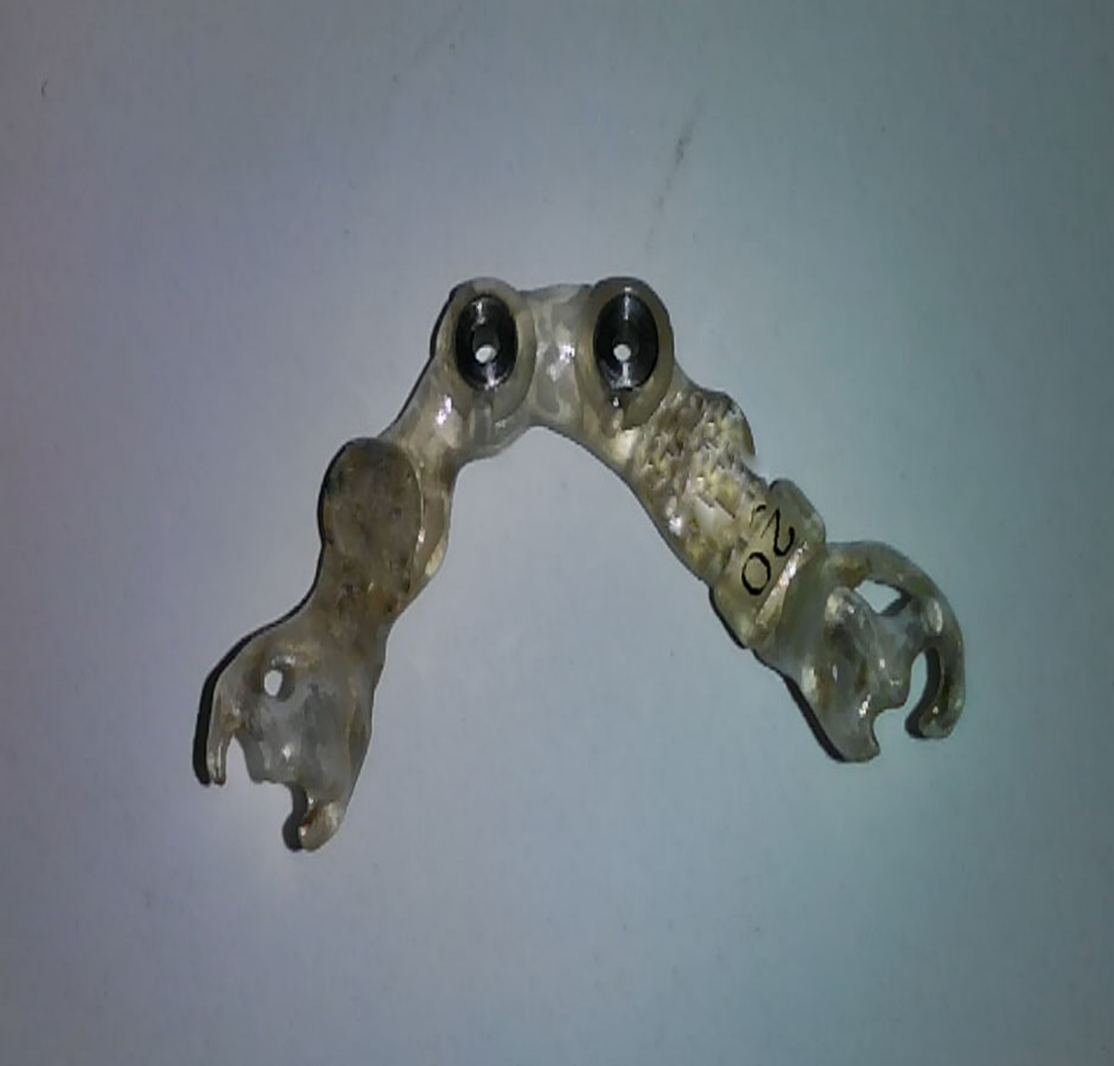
Surgical template fabricated using CBCT
